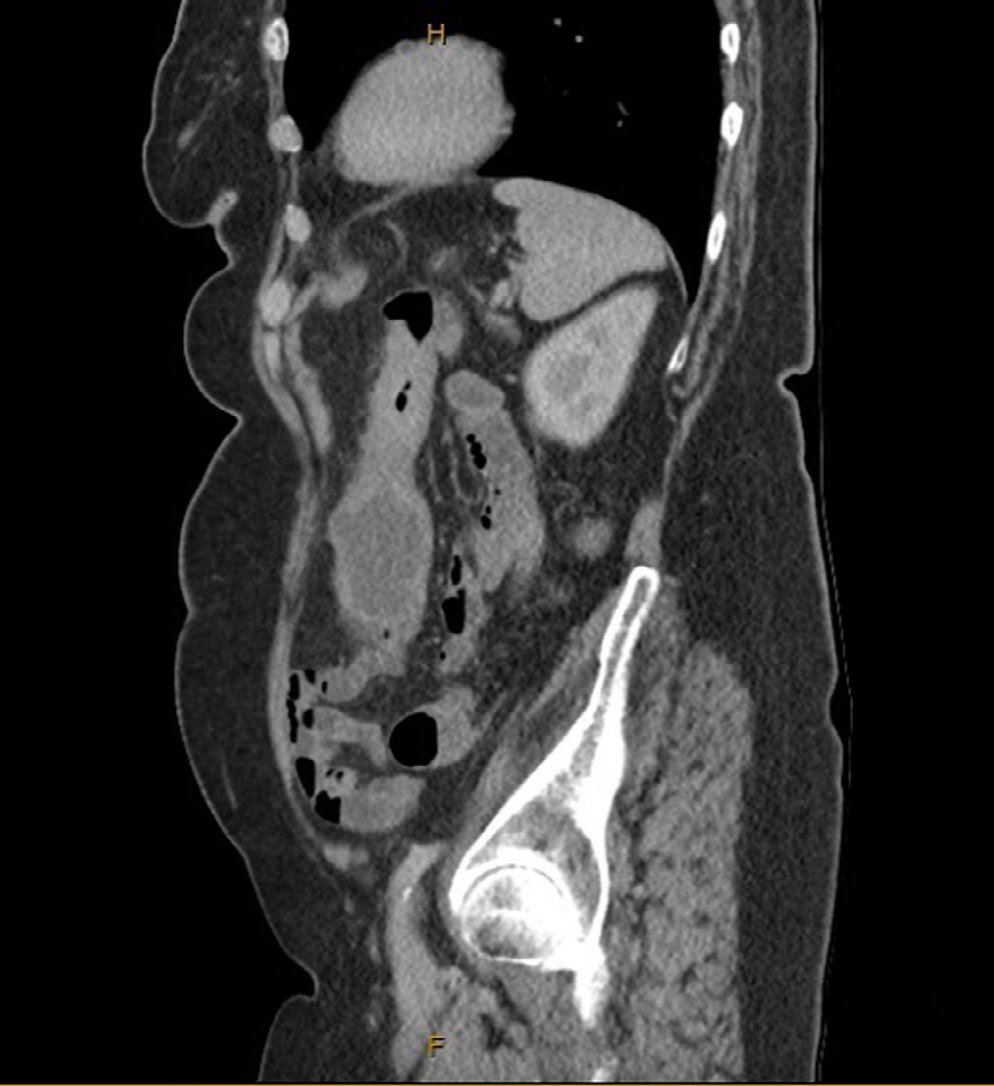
CT abdomen/pelvis coronal and sagittal view, respectively—long segment of concentric wall thickening and narrowing of the distal transverse colon with dilatation of proximal transverse colon and ascending colon
